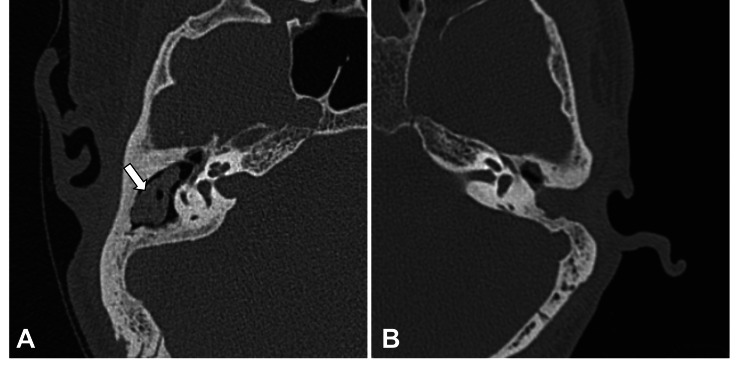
Axial right temporal HRCT image shows soft tissue density in the right post-operative mastoid cavity (A) suspected to be cholesteatoma was proven as wax (white arrow). Axial HRCT image of another patient showing soft tissue density in the left middle ear and post-operative mastoid cavity (B), which was proven to be granulation tissue. HRCT: high-resolution computed tomography
